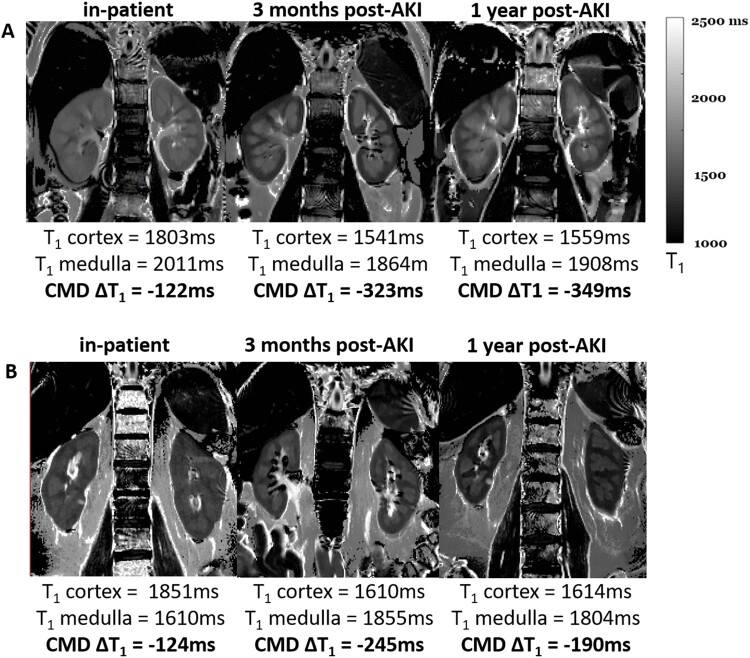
Example bFFE T_1_ maps through the long-axis of the kidney collected at the inpatient MRI scan and subsequently at 3 months and 1 year post-AKI. There is a clear lack of CMD for the inpatient T_1_ map, with T_1_ values of both the renal cortex and medulla being long. A reduction in the T_1_ of renal cortex and medulla is seen at 3 months and 1 year post-AKI, together with enhanced CMD. Data shown for (A) Subject 2 and (B) Subject 5, who had a significant drop in TKV.
